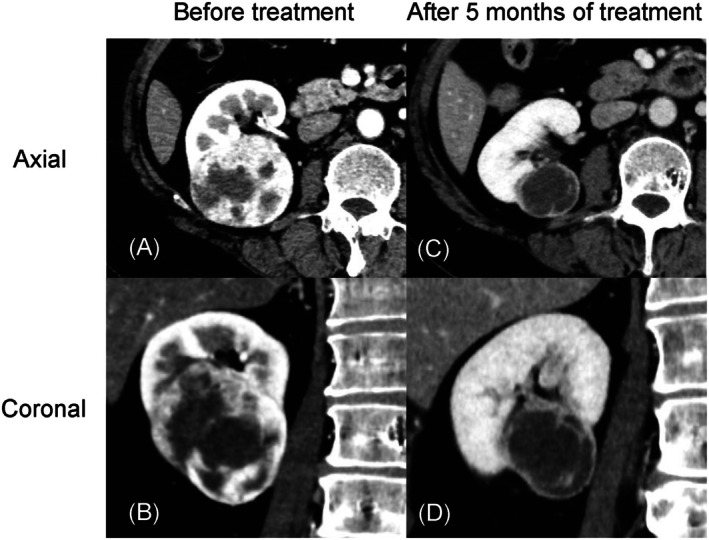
Contrast‐enhanced computed tomography images of the right kidney. (A) Axial view before treatment. (B) Coronal view before treatment. (C) Axial view after 5 months of treatment. (D) Coronal view after 5 months of treatment.
